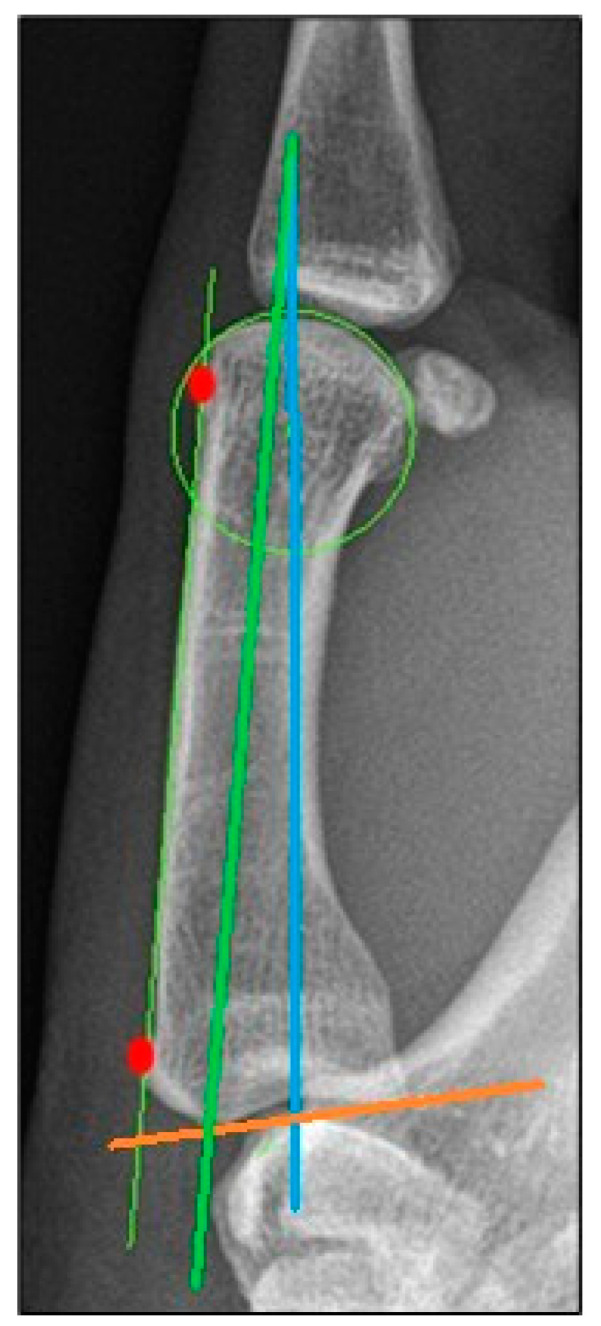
Determination of the axes in lateral conventional radiographs.
